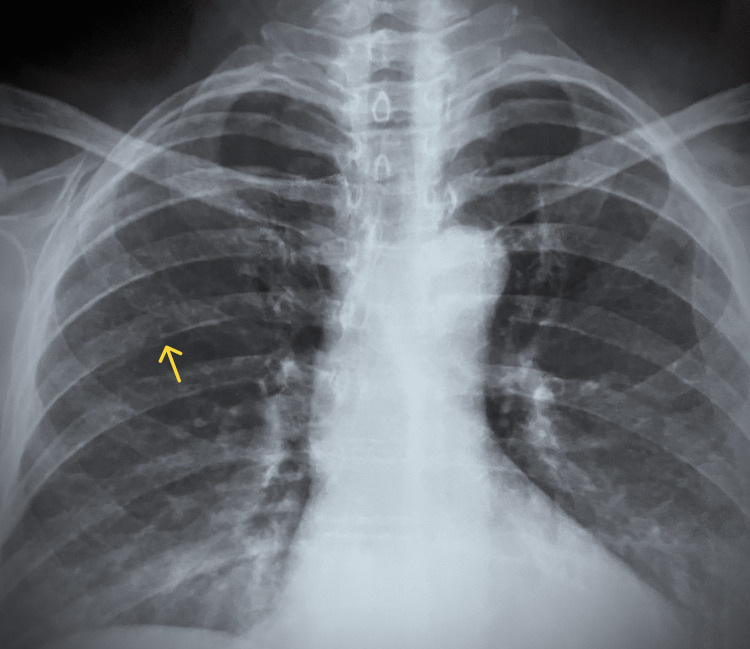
Chest X-ray of the patient showing clear lung fields with a rib fracture indicated by a yellow arrow on the right side. The cardiothoracic ratio and hila appear normal.
